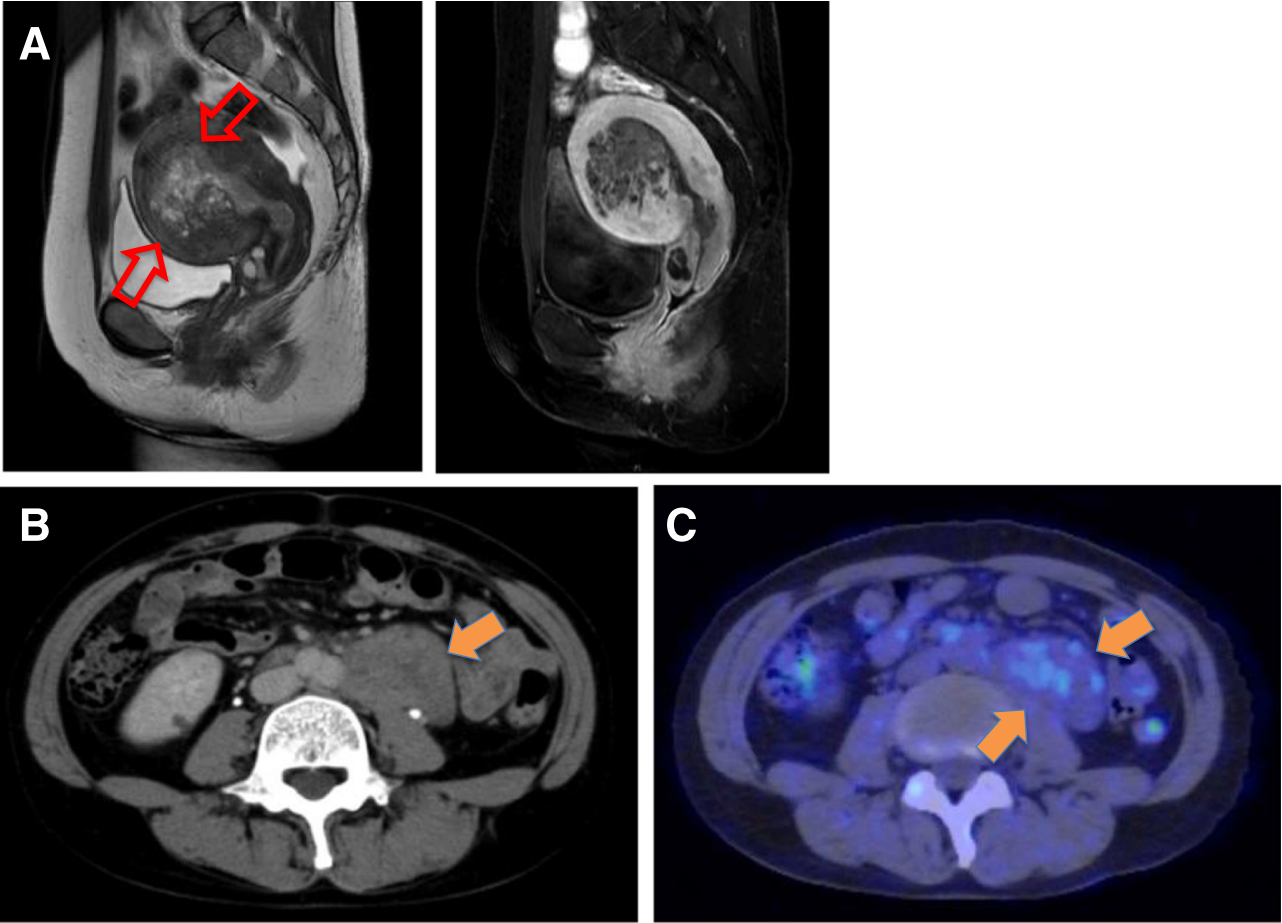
a Axial preoperative T2-weighted contrast magnetic resonance imaging (MRI) shows endometrium thickness in the uterine body. It has been diagnosed as Endometrial cancer of stage IB due to more than 50 % depth of myometrial invasion (*red arrowhead*). b The positron emission tomographic (PET) with CT scan. c shows pathologically elevated glucose metabolism in enlarged paraaortic lymph nodes (*orange arrowhead*)
